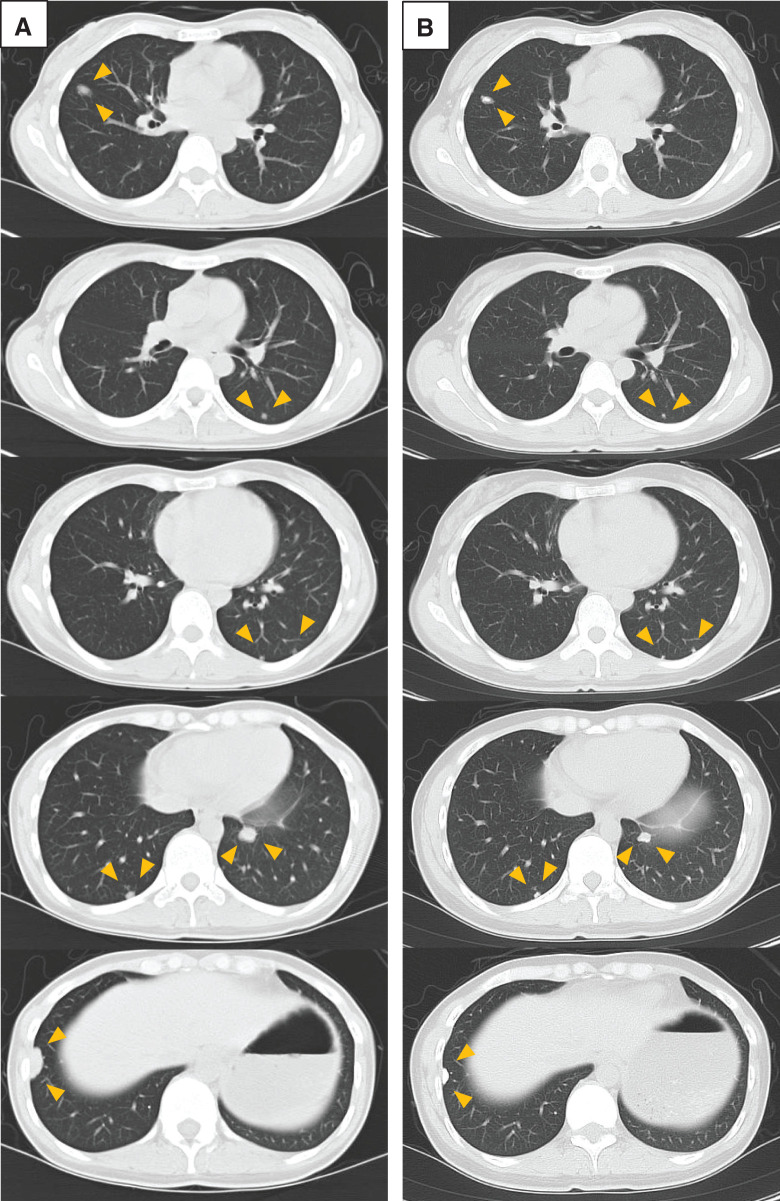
Serial chest CT findings before and after denosumab therapy. (A) Chest CT obtained before initiation of denosumab showing multiple, predominantly peripheral bilateral pulmonary nodules consistent with metastatic GCTB. (B) Chest CT after approximately 2.5 years of denosumab treatment demonstrating shrinkage, calcification, and stabilization of the nodules prior to metastasectomy. CT: computed tomography; GCTB: giant cell tumor of bone
